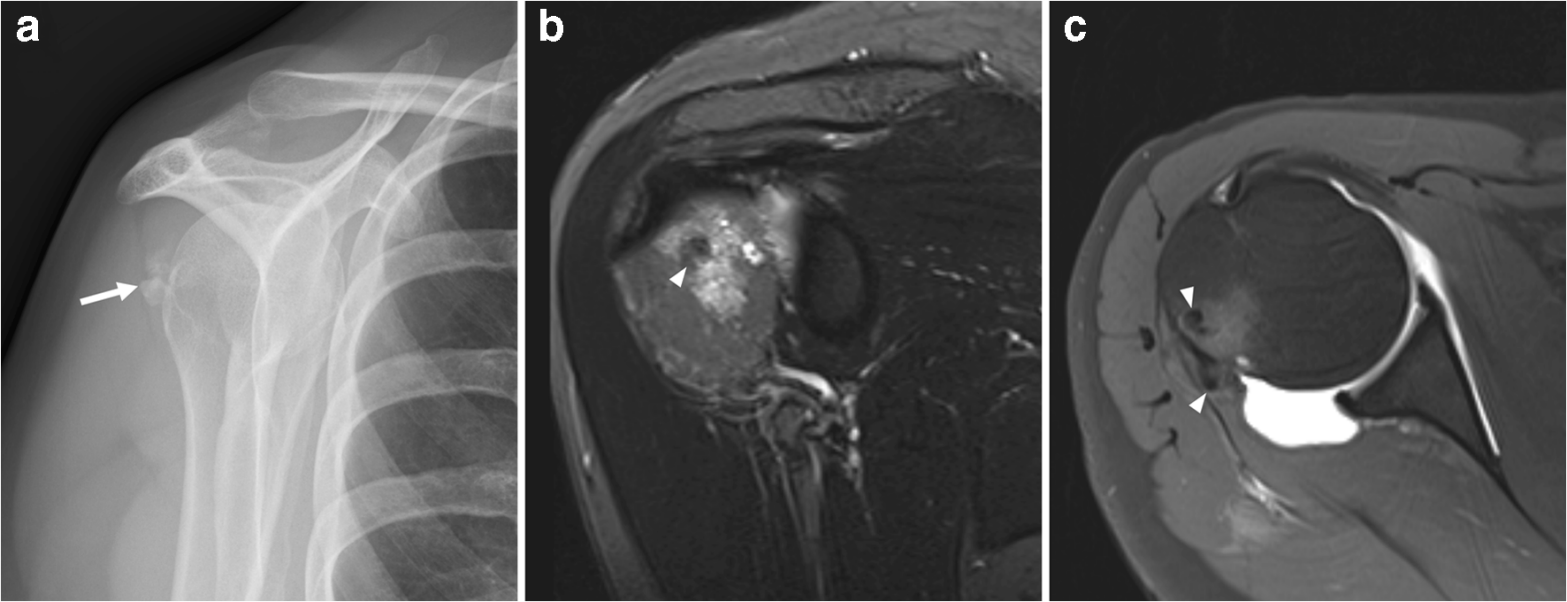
A 53-year-old woman with calcific tendinopathy of the infraspinatus tendon with osseous involvement. a Radiography shows soft tissue calcifications posteriorly to the humeral head. b Coronal oblique turbo inversion recovery magnitude image shows intra-osseous extension of calcification (*arrowhead*) and surrounding bone marrow edema. c Axial T1-weighted image with fat suppression after intra-articular injection of gadolinium shows calcifications which are located in the infraspinatus tendon and in the humeral head (*arrowheads*). There is no associated soft tissue mass
